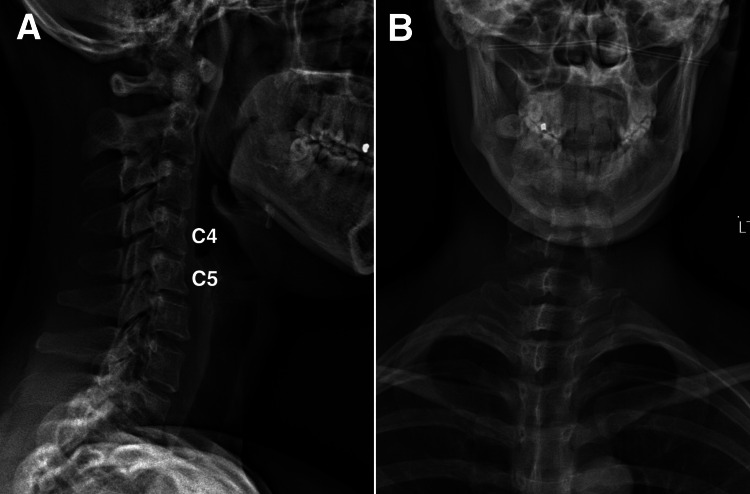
Radiograph of the cervical spine A) Reduced cervical lordosis and narrowing of the C4/5 disc were identified. B) Dextro-convexity at the lower cervical and thoracic regions was observed.
